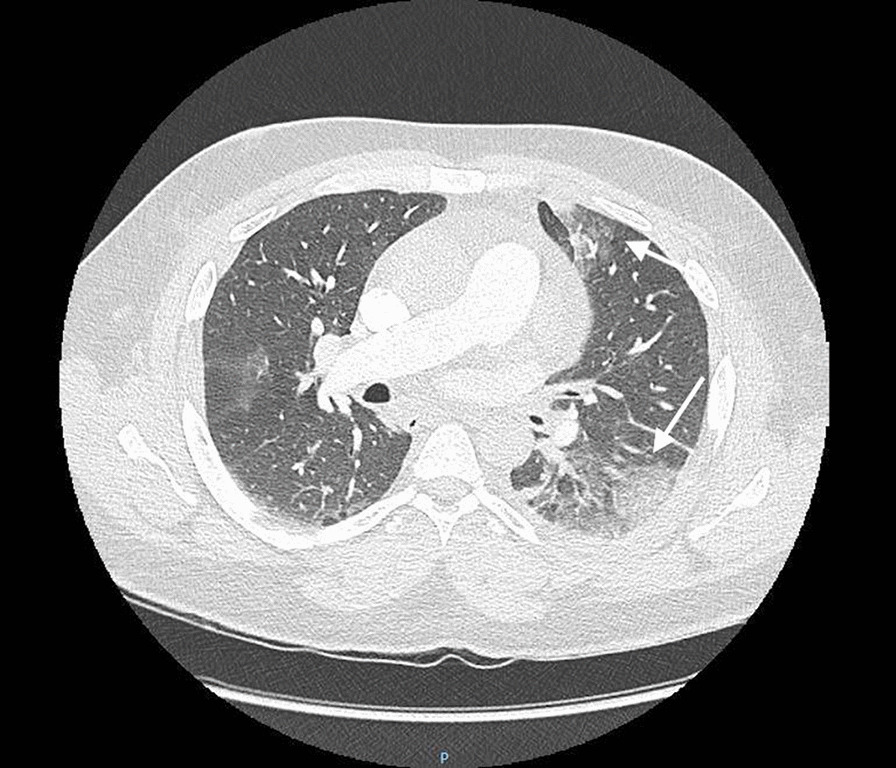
Computed tomography (CT) of the pulmonary parenchyma shows associated infarctions corresponding with ischemic lesions
